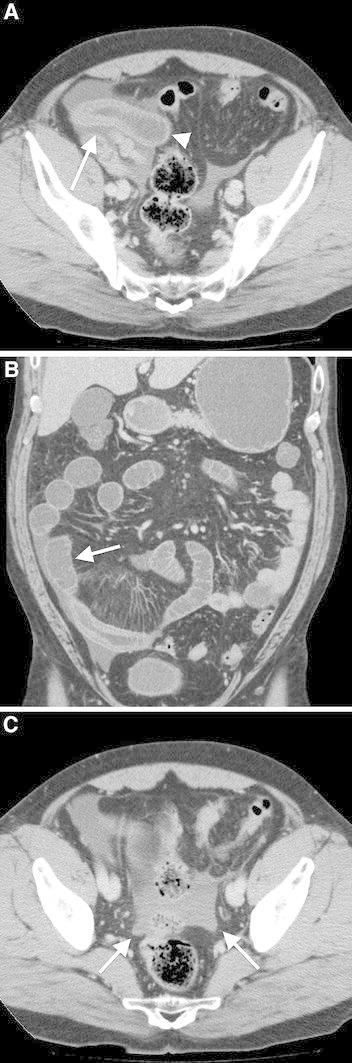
Intestinal anisakiasis in a 50-year-old man who presented with severe abdominal pain and a clinical history of eating raw fish 2 days before symptom onset. A CT shows severe, regional, submucosal edema of the small intestine (*arrow*). The proximal small bowel is dilated and fluid-filled (*arrow head*). B The distal small intestine of the involved intestinal tract is dilated with fluid collection (*arrow*). C The patient had ascites in the pelvis (*arrow*).
